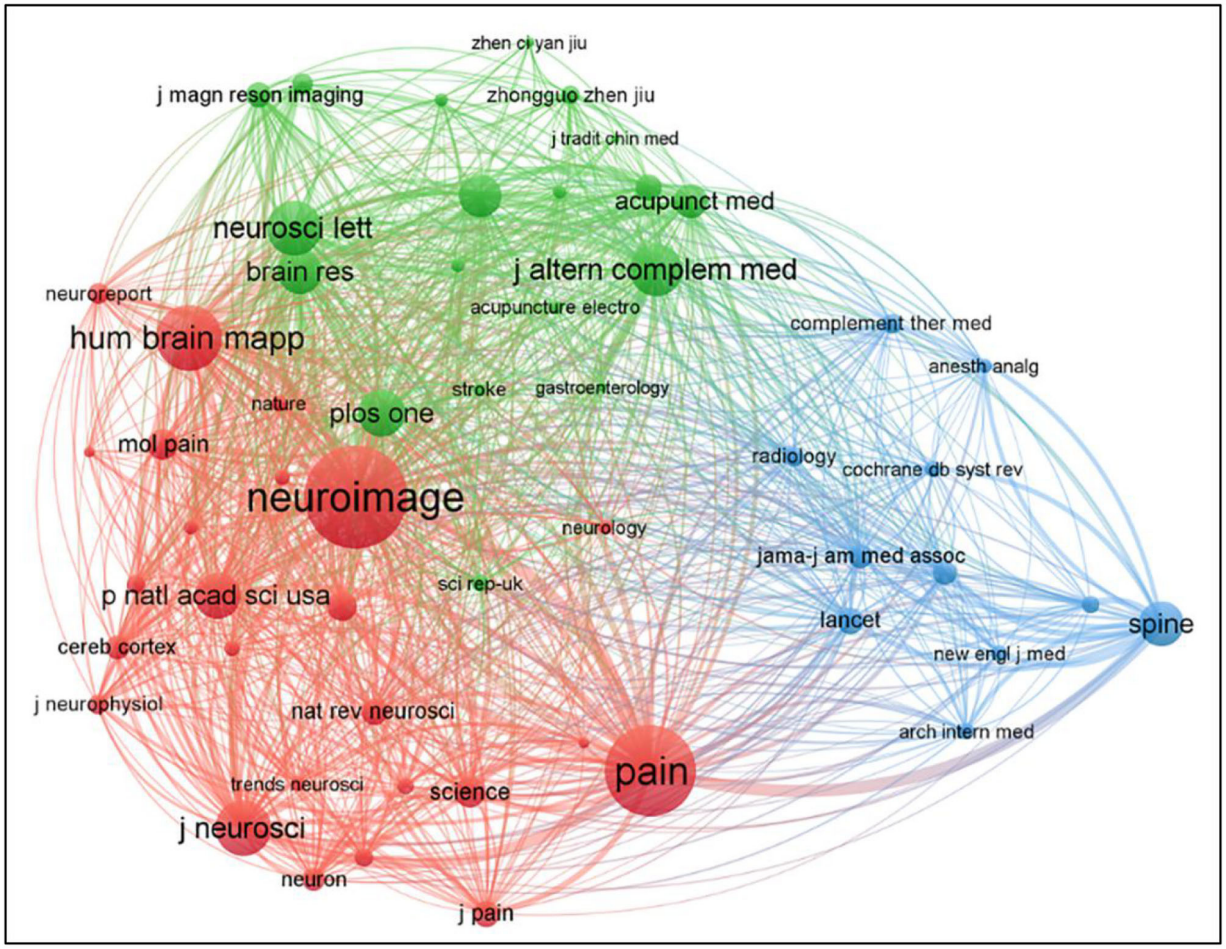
Map of cited journals on MRI research on acupuncture.
